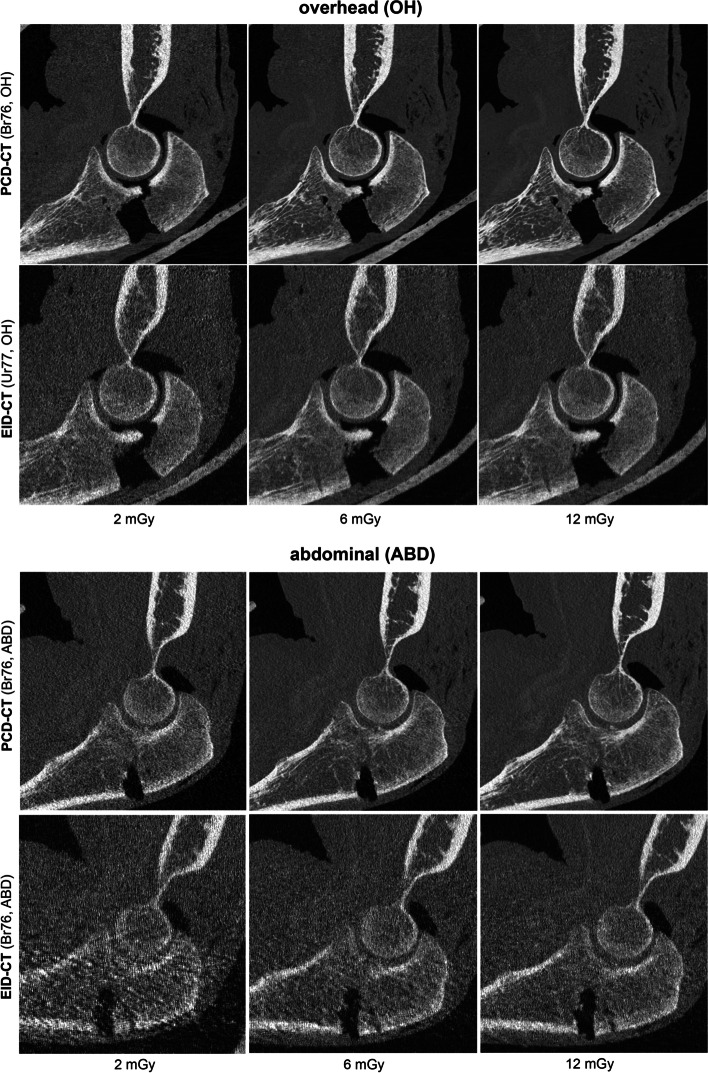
Comparison of photon counting detector (PCD)-CT and energy integrating detector (EID)-CT scans in overhead (OH) and abdominal (ABD) arm positions with Br76/Ur77 reconstruction kernels for low dose (2 mGy), standard dose (6 mGy), and high dose (12 mGy) scans
